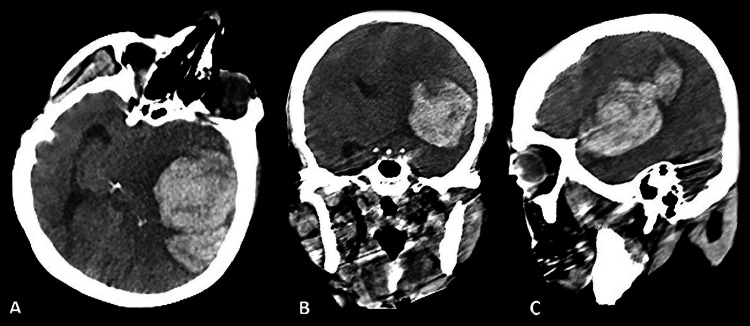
Preoperative Non-Contrast Enhanced Computed Tomography A: Preoperative cranial computed tomography axial scan showing a 6x6 cm hyperdense area in the left temporal lobe extending into the intraventricular space, with perilesional edema detected. B: Preoperative cranial computed tomography coronal scan revealing obliterated major sulci and a faint left lateral ventricle. A mass effect with a 9 mm midline shift was observed. C: Preoperative cranial computed tomography sagittal scan displaying a 6x6 cm hyperdense area, consistent with hemorrhage and perilesional edema detected.
